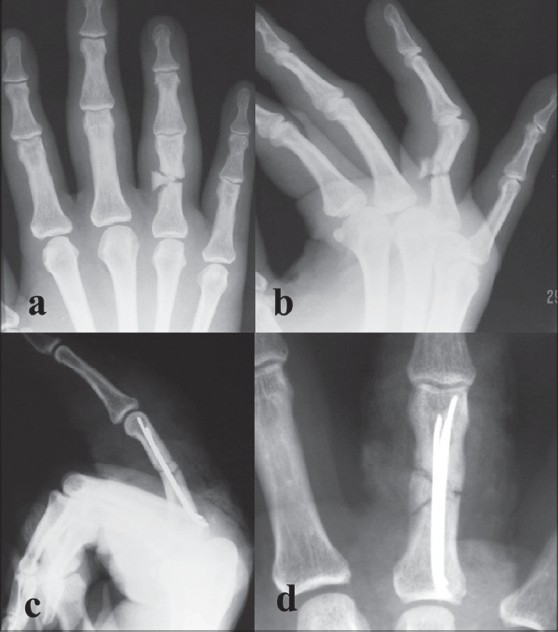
(a) AP view (b) lateral view of left hand shows unstable segmental proximal phalangeal fracture. Postoperative AP and lateral X-ray (c and d) of left hand of same patient shows segmental fracture fixed with multiple nails
